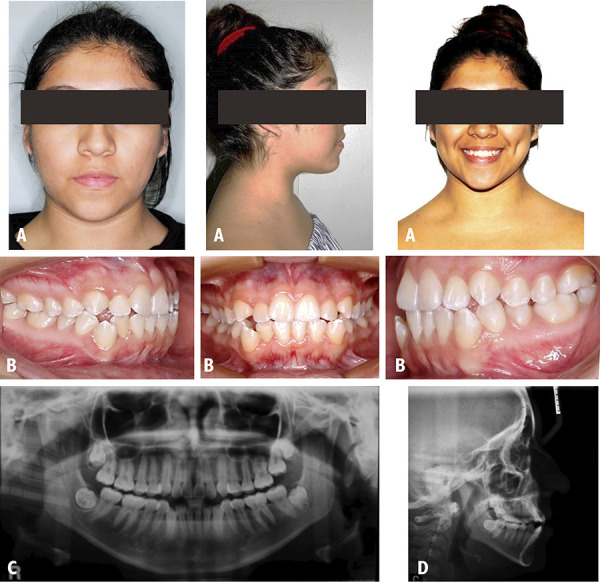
Case 1. Initial pre-treatment records. A: Extraoral photographs. B: Intraoral photographs. C: Panoramic radiograph. D: Cephalometric radiograph.
